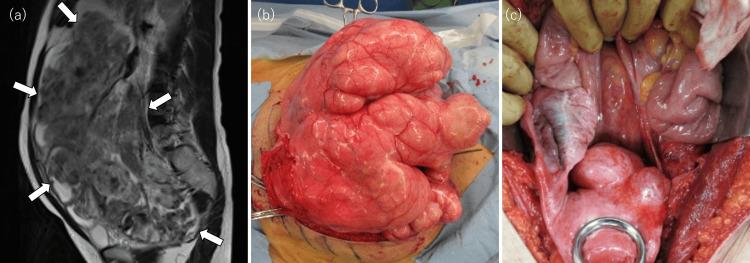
Findings from the previous hospital (a) MRI revealed a large pelvic mass (30cm) arising from the posterior wall of the uterus. (White arrows delineate the margins of the tumor.) (b) Intraoperative findings at the start of first surgery. The exophytic multinodular mass was arising from the uterine fundus. (c) Intraoperative findings at the end of first surgery. There was 8cm of remaining tumor arising from the posterior wall of uterus.
